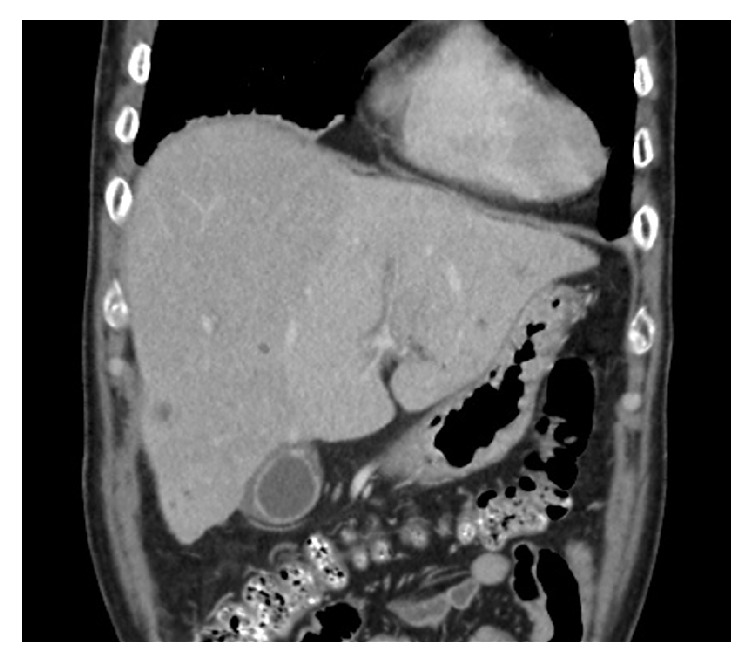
Coronal computed tomography (CT) scan (portal venous phase) shows gallbladder wall congestion and the periportal halo is visualized in the left lobe of the liver.
